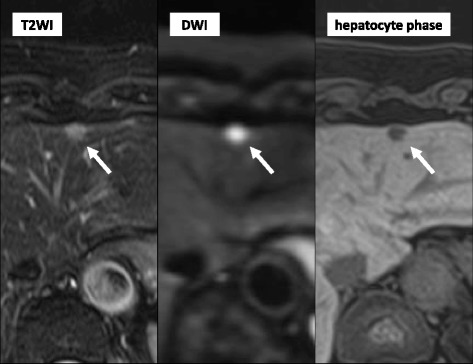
Magnetic resonance imaging (MRI) showing a nodule with slight high intensity on a T2-weighted image and high intensity on a diffusion-weighted image. Gadolinium-ethoxybenzyl-diethylenetriamine pentaacetic acid-enhanced dynamic MRI showing a nodule with defects in the hepatocyte phase in segments 2, 3 (*arrow*), 4, and 8 of the liver
